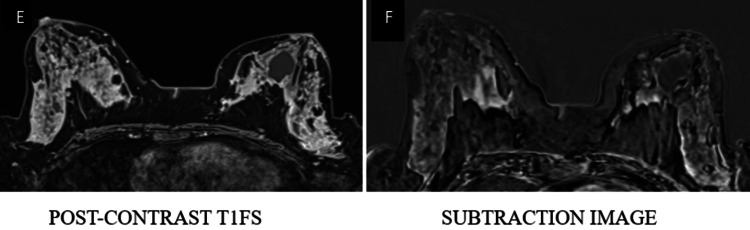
Post-contrast T1FS and subtraction image (E, F) demonstrate no post-contrast enhancement T1FS: T1-weighted with fat saturation
